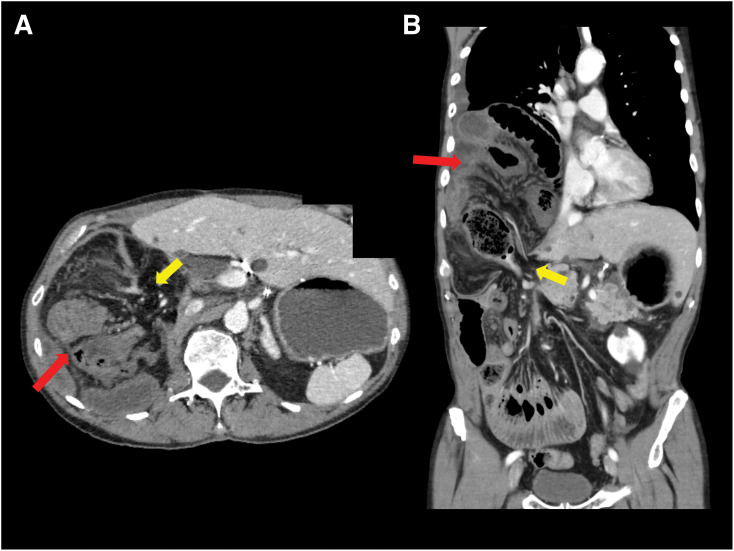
CT scan from the referring hospital. The yellow arrows denote the defect in the right diaphragm, and red arrowheads indicate the herniated ascending colon and small intestine within the right thoracic cavity. (A) Axial slice. (B) Coronal slice.
